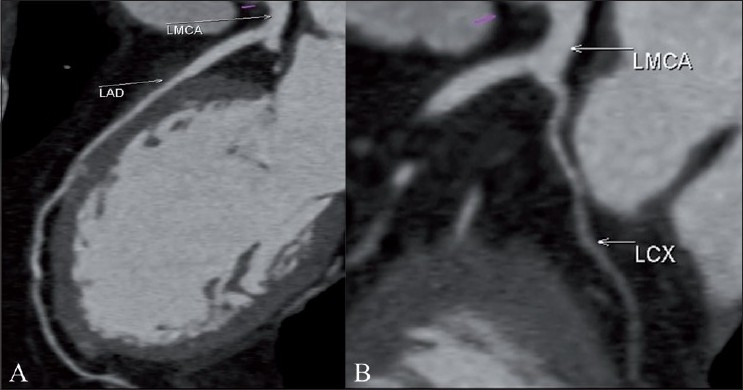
Cardiac CT. The curved MPR images show the origin and course of the left main coronary (LMCA), left anterior descending (LAD) (A), and left circumflex artery (LCX) (B)
